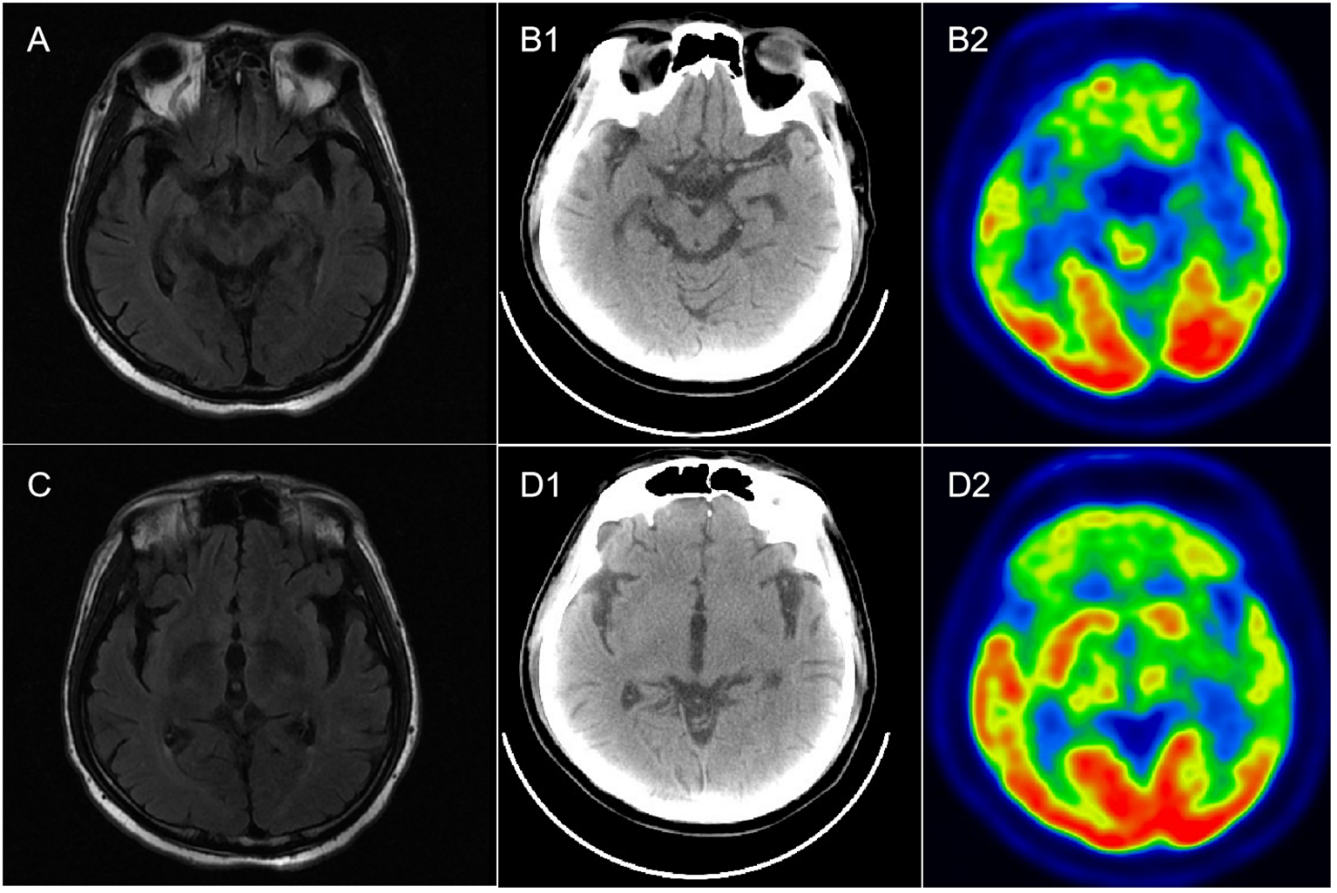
Brain MR and 18F-FDG PET-CT imaging in a patient with anti-DPPX encephalitis. (A, C) Mild atrophy of the hippocampus and temporal lobe in T2-flair imaging. (B1, B2) Normal metabolism in both hippocampus in PET-CT. (D1, D2) Hypometabolism in the bilateral frontal lobes, parietal lobes, and temporal lobes, especially the left side.
